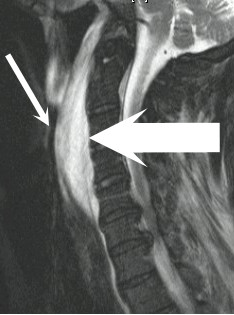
Sagittal T2 weighted MRI with fat saturation techniques revealing marked increase in the prevertebral soft tissue prominence secondary to an enlarging hematoma (large arrow). Note the severe compromise of the oropharyngeal airway (small arrow).
